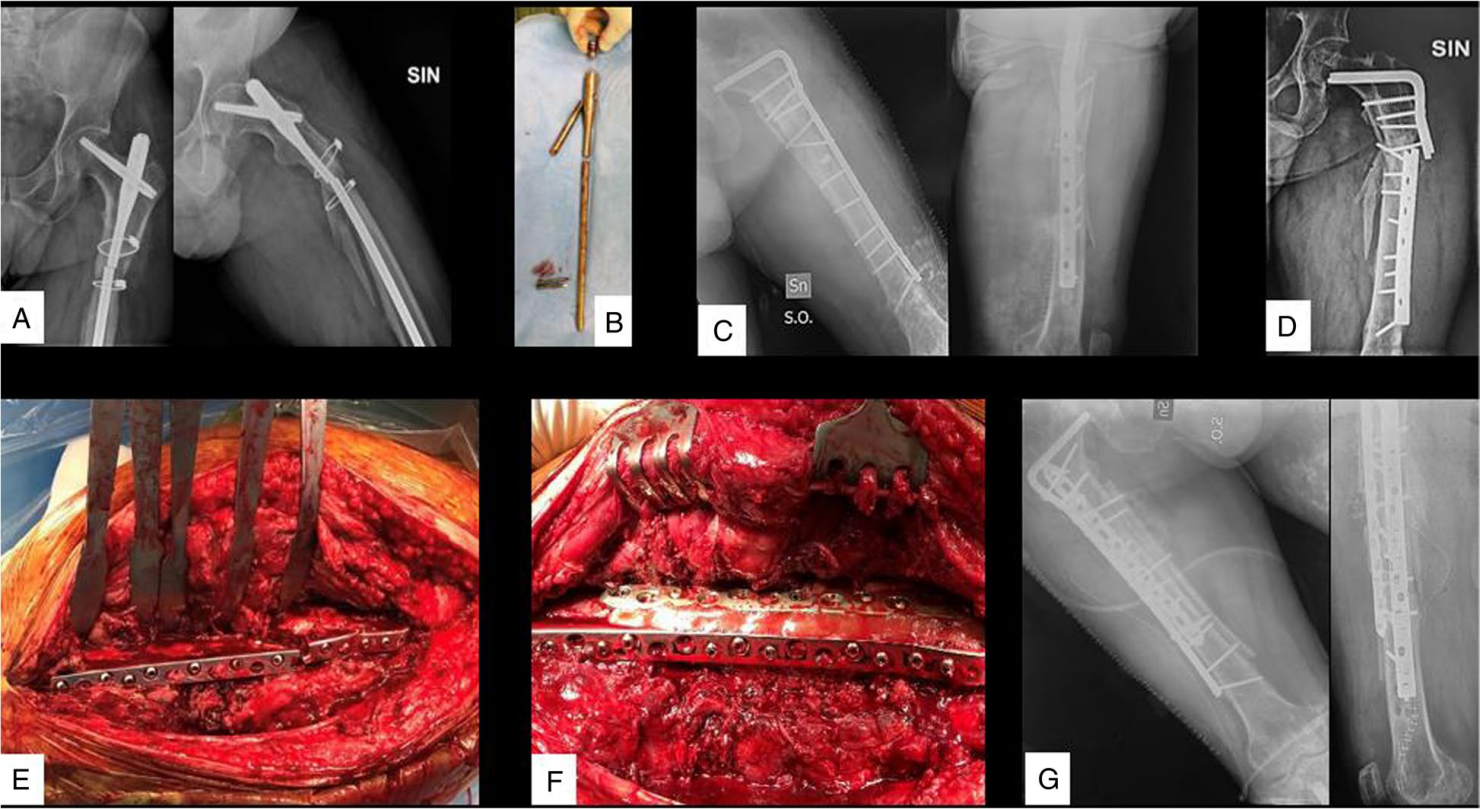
A AP and LL radiographs of the femur, showing a subtrochanteric non-union with a broken EM nail, at 1 year after the primary surgery; B intra-operative image of the broken EM nail; C AP and LL radiographs of the first reintervention with 95°blade plate, medial strut allograft and RIA autograft; D blade plate failure, at 3 months follow-up; E intraoperative image of the broken blade plate; F intraoperative image with the double-plating revision technique and medial strut allograft; G AP and LL radiographs of the second reintervention
